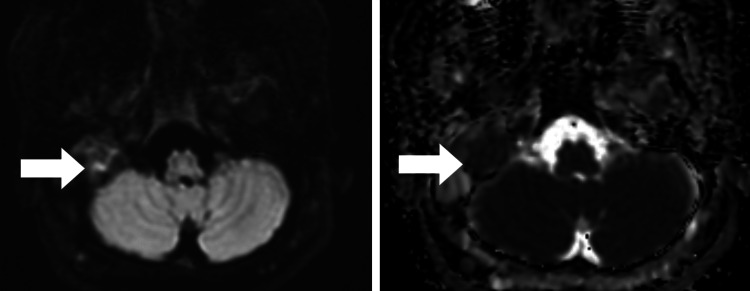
An axial diffusion-weighted MR image showing a few areas of diffusion hyperintensity in the right middle ear cavity and mastoid air cells associated with a signal drop on the ADC images. ADC: apparent diffusion coefficient, MR: magnetic resonance.
